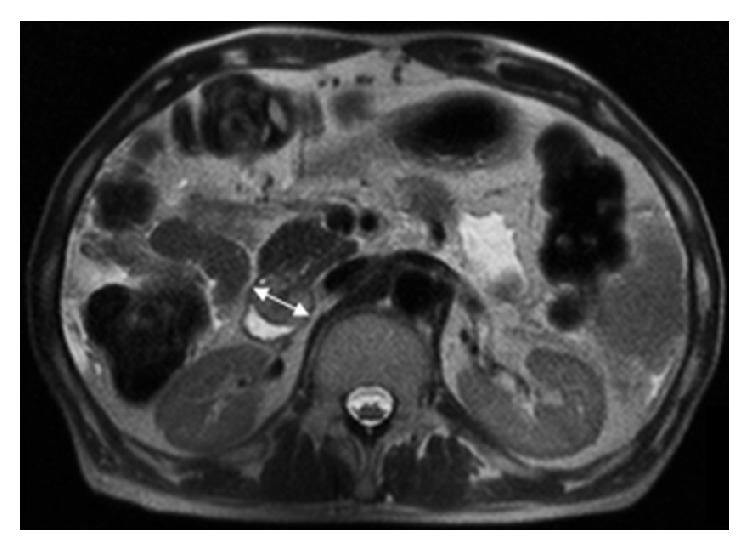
Abdominal magnetic resonance imaging shows a pedunculated mass at the level of ampulla with mild enhancement (double arrow).
